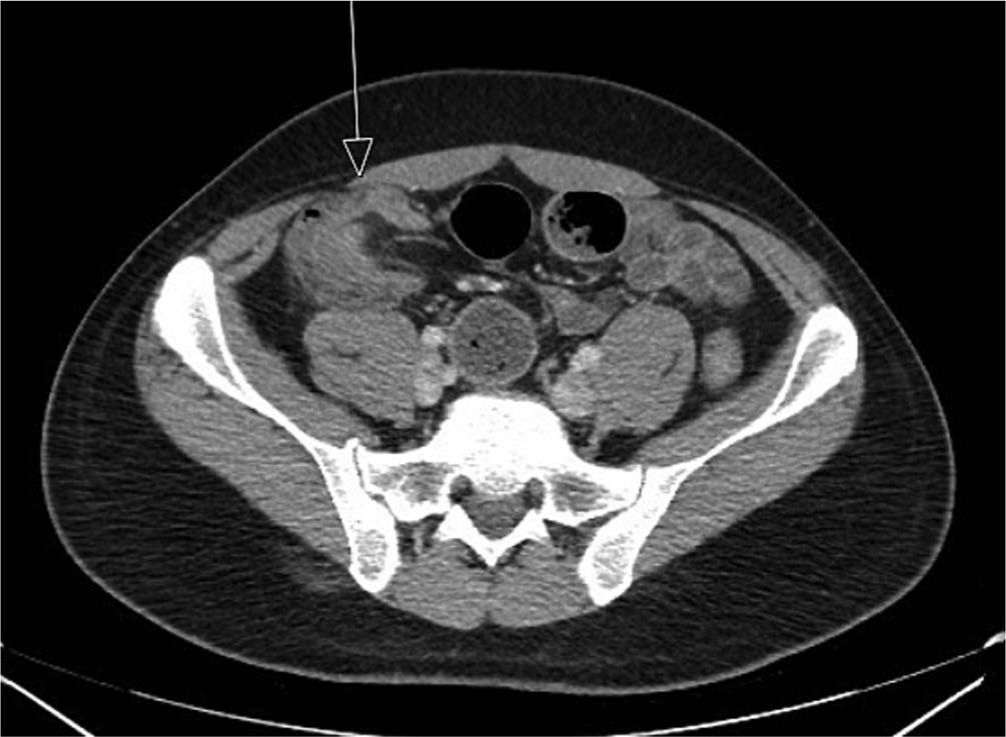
Initial CT imaging, coronal cut, showed an abnormal ileocolic configuration with intussusception of the distal ileal loop through the cecum.
